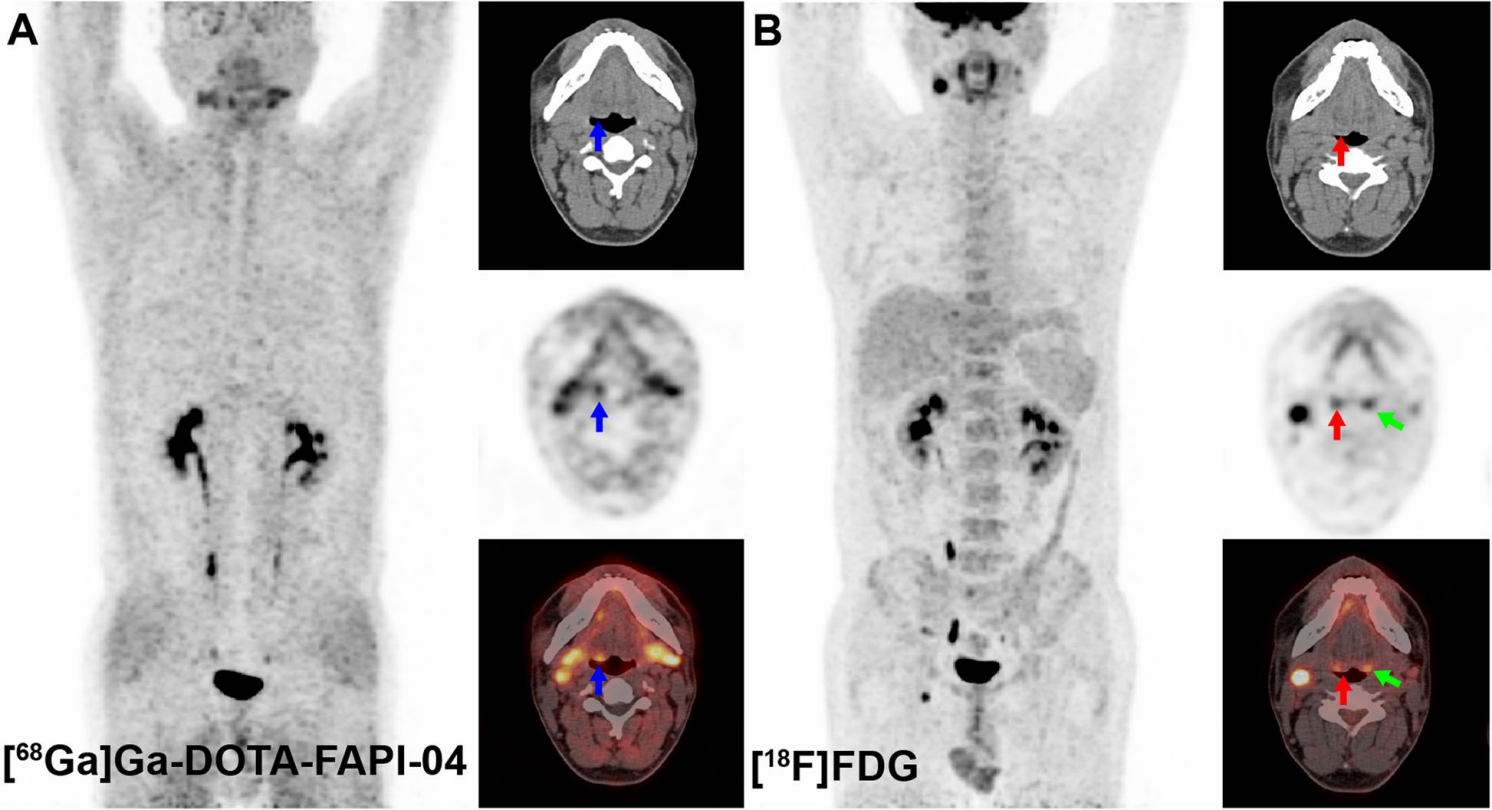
[^68^ Ga]Ga-DOTA-FAPI-04 PET/CT (A) and [^18^F]FDG PET/CT (B) images of a 39-year-old man with tonsil cancer. [^68^ Ga]Ga-DOTA-FAPI-04 showed high uptake in the primary lesion (blue arrows; SUV 1.53) and no physiological uptake in the contralateral tonsil. [.^18^F]FDG showed lower uptake in the primary lesion (red arrows; SUVmax 3.76) and higher physiological uptake in the contralateral tonsil than in the primary lesion (green arrows; SUVmax 5.76)
